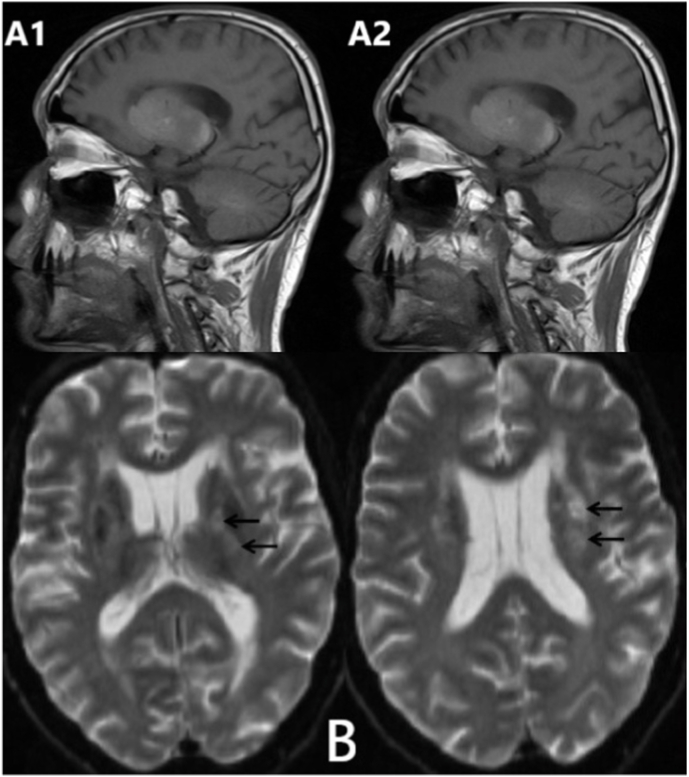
Brain MRI; (A); High-signal intensity lesions on T1-weighted consist with symmetric calcifications of the right (A1) and left (A2) basal ganglia.(B); High-signal intensity lesions on diffusion-weighted imaging consist with lacunar infarcts in the left caudate nucleus and corona radiate (black arrows).
